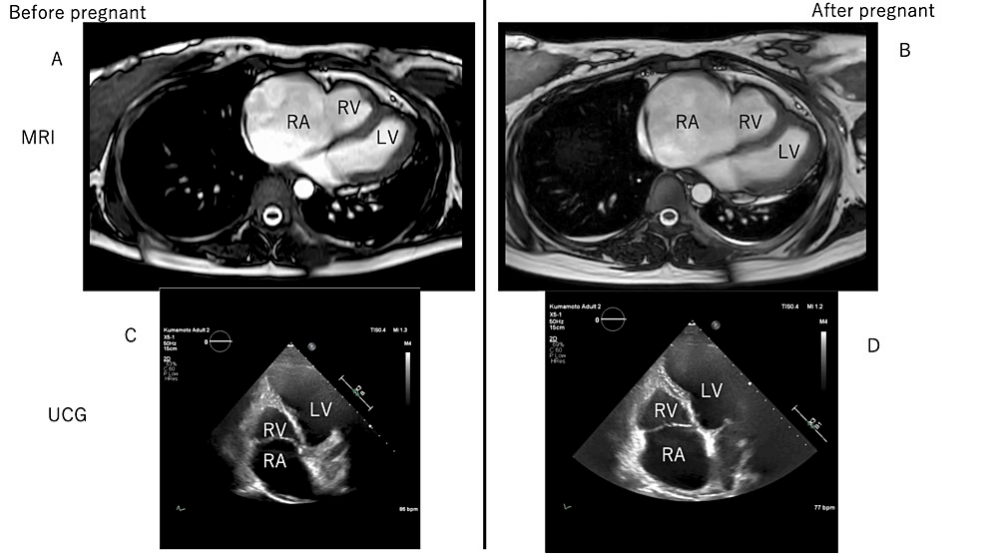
Right heart changes before and after pregnancy in magnetic resonance images (MRI) and transthoracic echocardiography (TTE) LV: left ventricle RA: right atrium RV: right ventricle Panel A: before pregnancy MRI image   Panel B: after pregnancy MRI image Panel C: before pregnancy TTE image     Panel D: after pregnancy TTE image
